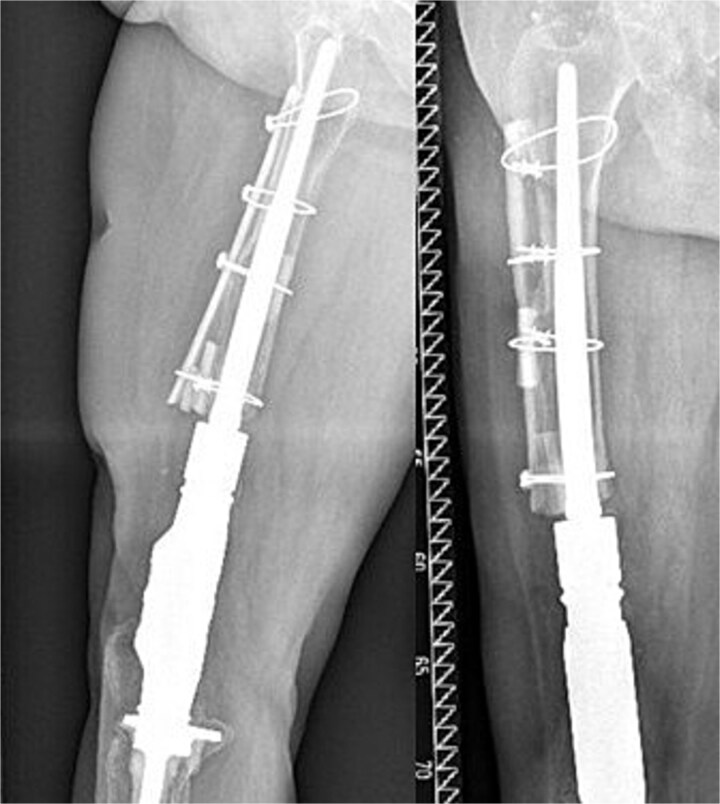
Postoperative two-view radiographs following reconstruction with a cortical strut graft and modular knee arthrodesis.
